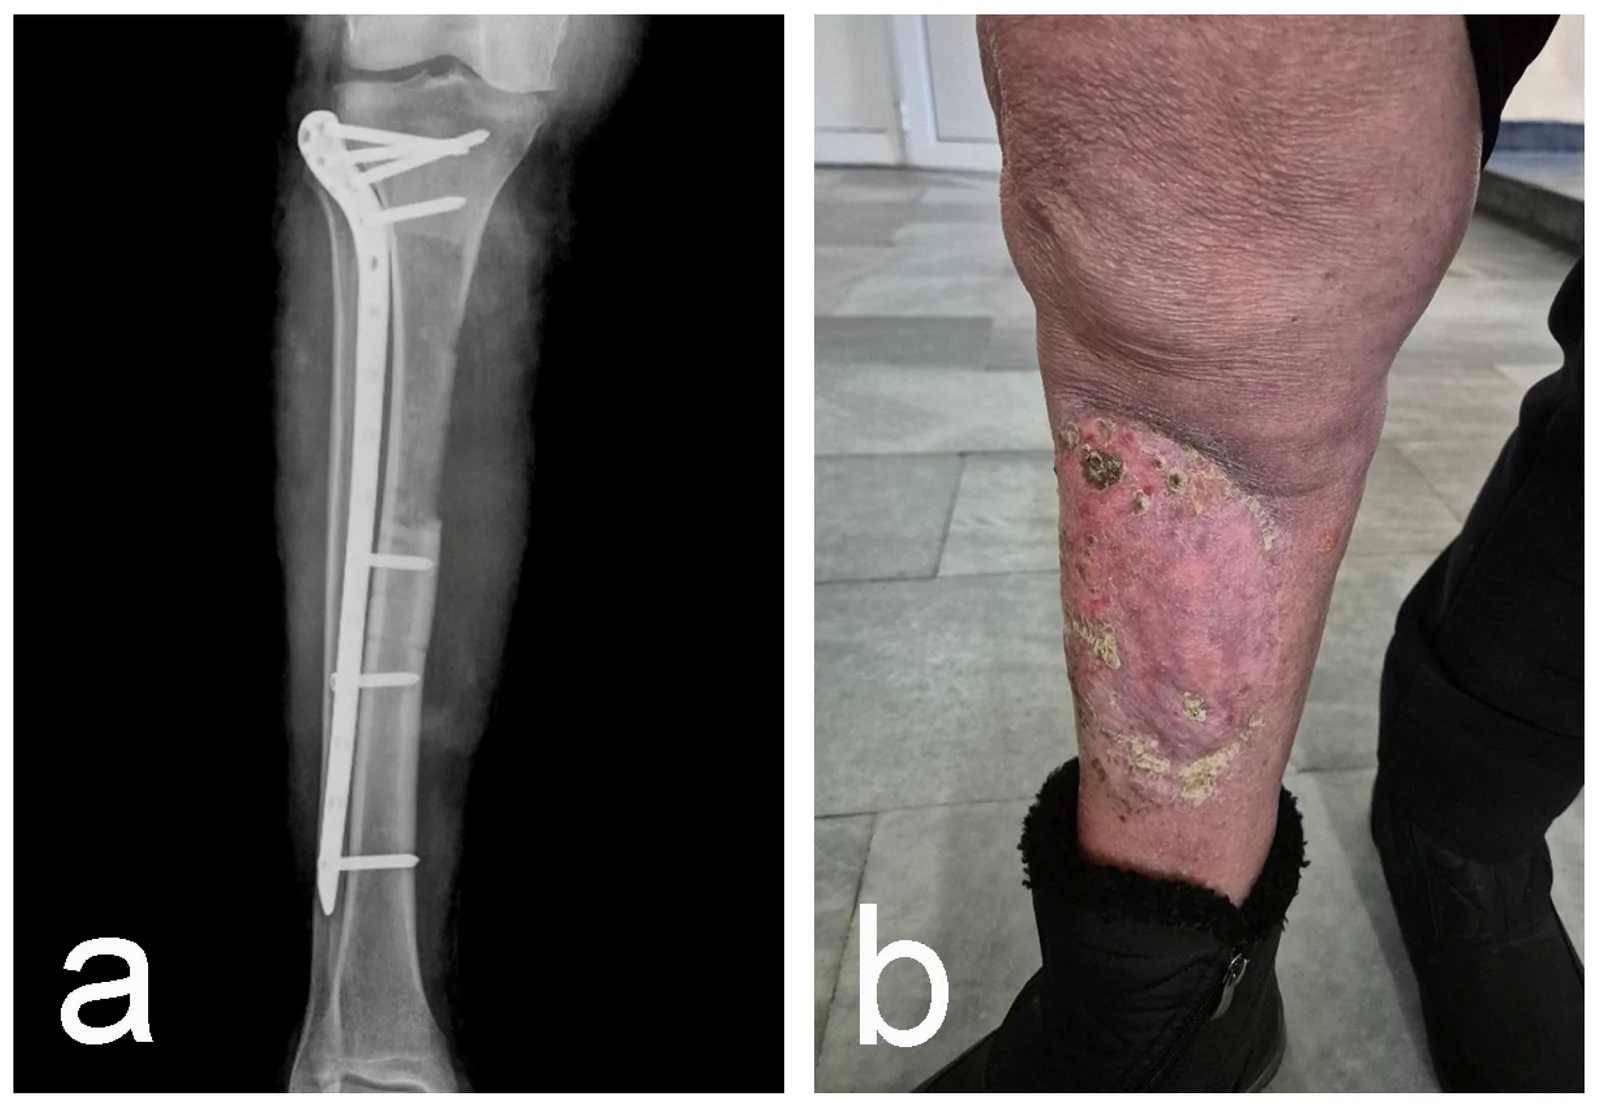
Postoperative imaging and clinical outcome at approximately 16 months. (a) Anteroposterior radiograph demonstrating the prophylactic locking tibial plate. (b) Clinical photograph showing stable coverage by the gastrocnemius muscle flap and free skin graft without wound breakdown or hardware exposure.
